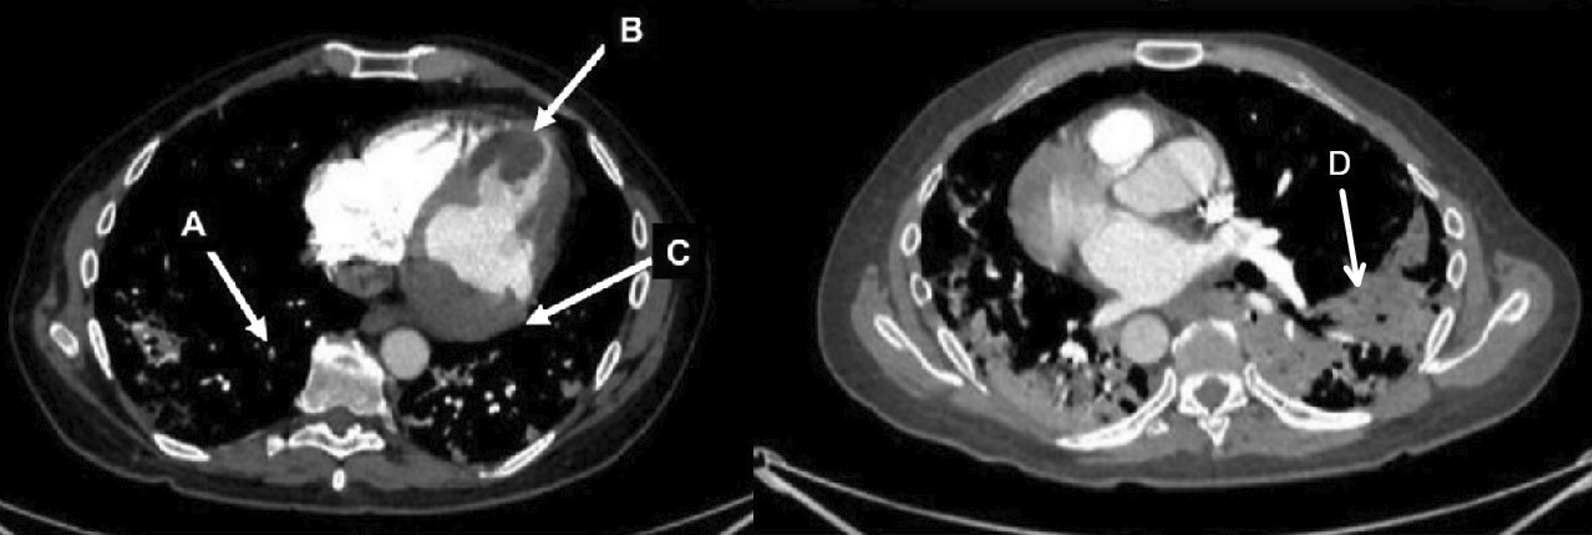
Case 8. Figure 3. CT scan of the chest. Contrast axial view showing a sub-segmental pulmonary embolism of the right lower lobe (A), a mass near in the inferior border of the heart B and in LV apex (C), and multifocal pulmonary infiltrates (D)
